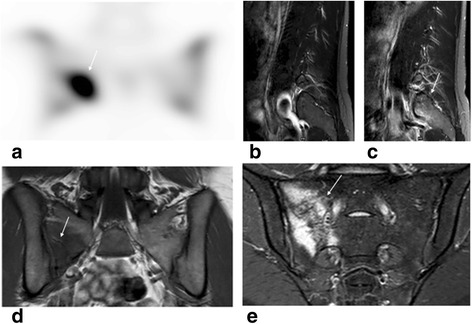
Twenty-two-year-old female long distance runner with sacral stress fracture which initially mimicked radicular pain (lower lumbar pain with pain/numbness in buttock) and later the pain was localized over the right sacroiliac joint. a Coronal SPECT image of the pelvic region shows intense uptake in the right lateral aspect of the sacrum. b, c Sagittal T2 STIR MR sequences *left* and *right*, respectively, performed 1 month prior to bone scan for “radicular type lumbar pain” was read as negative, but coronal pelvic T2-weighted images were not obtained. In retrospect sagittal T2 STIR image right (c) showed edema in the right sacrum (seen at the edge of the field of view only). Repeat MRI of the pelvis 1 day after bone scan showed nondisplaced right lateral sacral fracture on coronal T1 weighted images (d) with associated bone marrow edema seen in coronal T2-weighted SPAIR image (e). Athletes with lower lumbar pain and negative lumbar spine MRI would benefit from additional coronal pelvis images to exclude referred lumbar pain caused by pelvic injuries
